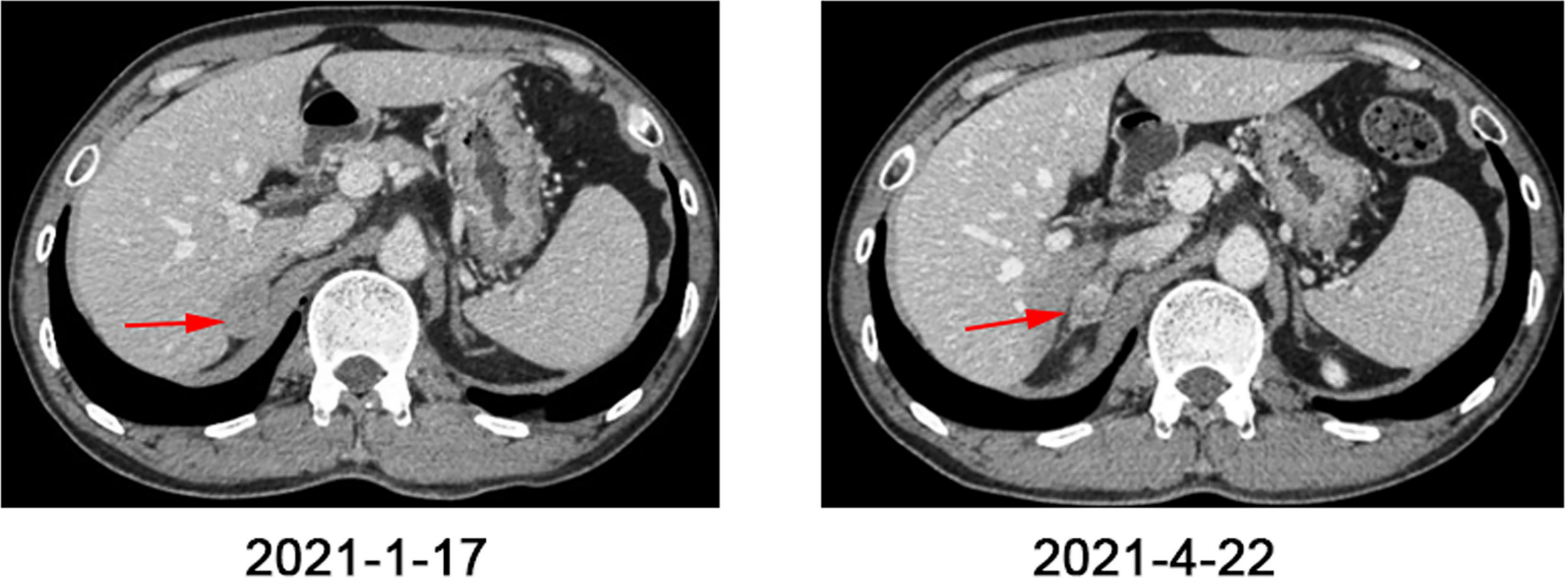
Abdomen CT scans before and after one cycle of triple-combination therapy and two cycles of sintilimab treatment.
